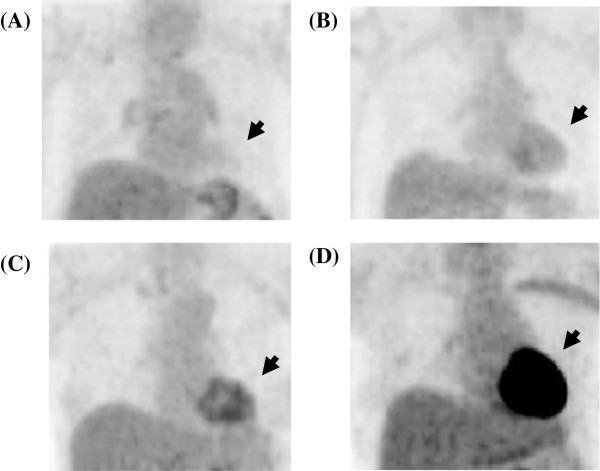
Visual evaluation of myocardial ^18^F-FDG uptake using a four-point scale. Myocardial ^18^F-FDG uptake was lower than hepatic uptake (A, grade 1), similar to hepatic uptake (B, grade 2), somewhat higher than hepatic uptake (C, grade 3), and noticeably higher than hepatic uptake (D, grade 4). Grade 1 can be recognized as a complete inhibition of physiological uptake.
